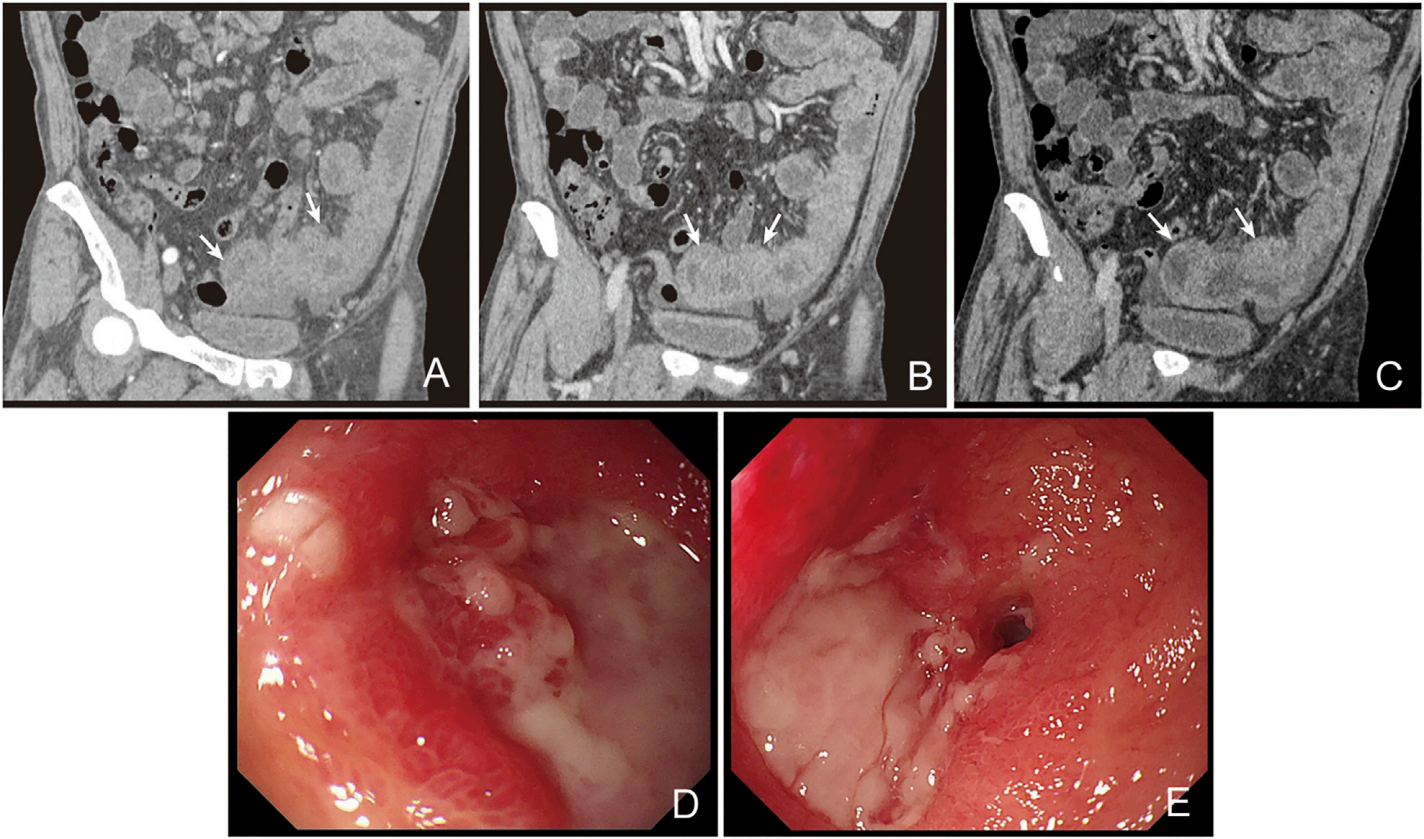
CT scan and enteroscope of the patient. CT enterograph of the small intestine showed even thickening in the wall of the small intestine (jejunum) in the pelvic cavity with multiple lymphadenopathy in enterocoelia and retroperitoneum ((A), arterial phase; (B), portal phase; (C), lag phase). Enteroscopic examination showed multiple ulcers in the distal jejunum (D). The ulcers were deeper with irregular margins and were covered with white pus moss. Enteroscopic examination showed stenosis in the jejunum (E).
